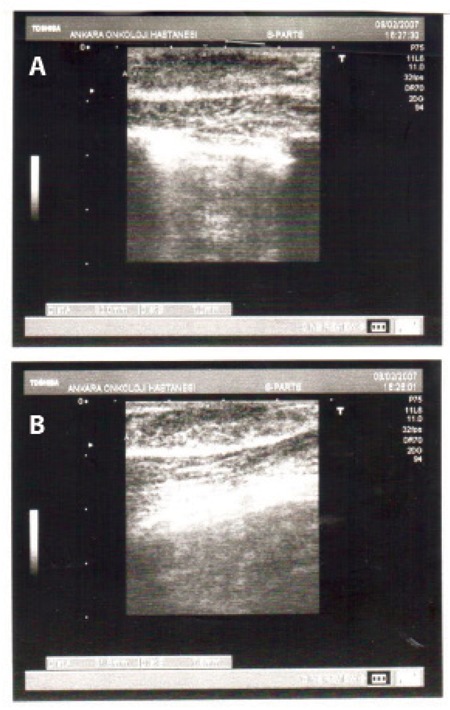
a) Breast tissue of 32x7.5 mm on the right side byultrasonography. b) Breast tissue of 30x7 mm on the left side
